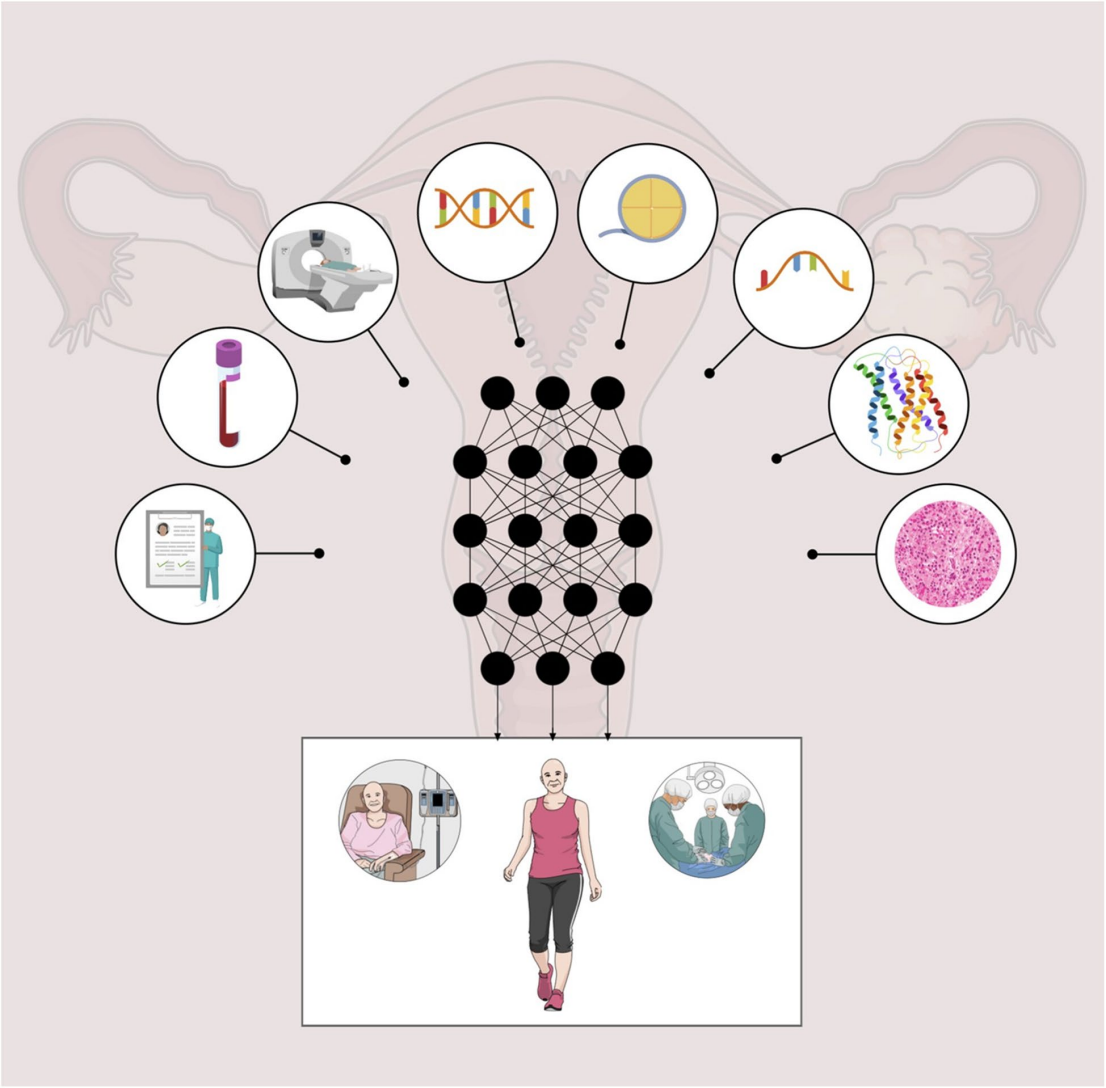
Multiomics studies in ovarian cancer to date use (circles from left to right) clinical data, serum biomarkers like cancer antigen 125 (CA-125), imaging including computed tomography (CT), magnetic resonance imaging (MRI), and ultrasound (US), genomics, epigenomics, transcriptomics, proteomics, and pathology data for the development of artificial intelligence-based cancer subtyping, lesion classifiers, and models for predicting patient outcome including response to chemotherapy, complete surgical cytoreduction, and survival (bottom panel)
